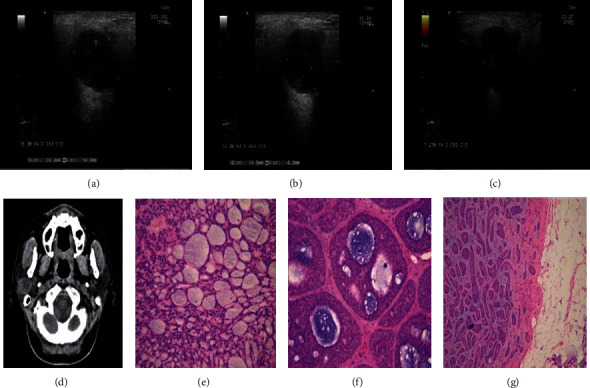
A 56-year-old woman of a solid-type BCA. (a, b) Transverse grayscale ultrasonographic image of a 56-year-old woman. An ill-defined, regular, mixed echo mass with heterogeneous internal architecture and with calcification without light posterior enhancement in the right parotid gland. (c) A small amount of blood on Doppler B. (d) Plain computed tomography scan showing a regular, ill-defined, uneven mass with low density and calcification. (e, f) (solid) Tumor cells are arranged in a sheet or island structure, and some are with heteromorphic; extensive cribriform structures are present (×200); Scar bar. (g) Tumors are invading the fat cells (×100).
